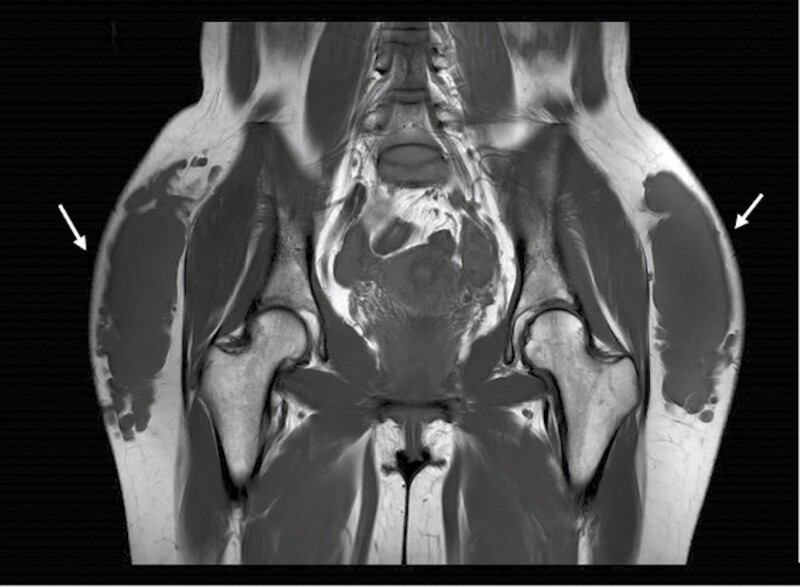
MRI scan of the gluteal region (coronal view): accumulation of poorly defined fillers in different fat layers of both buttocks (white arrows).
